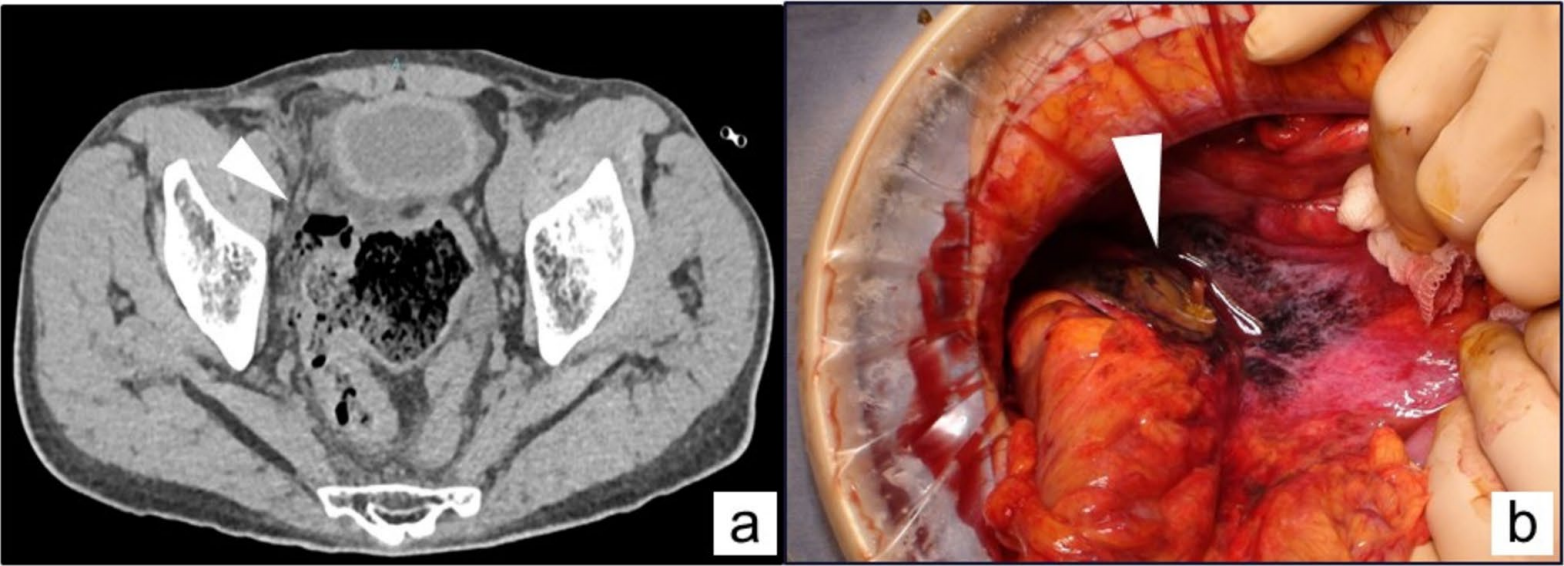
a Contrast-enhanced computed tomography showed free air around the sigmoid colon, as indicated by a triangular arrow. b Intraoperatively, the perforation of the sigmoid colon was identified, with the perforation indicated by the triangular arrow
